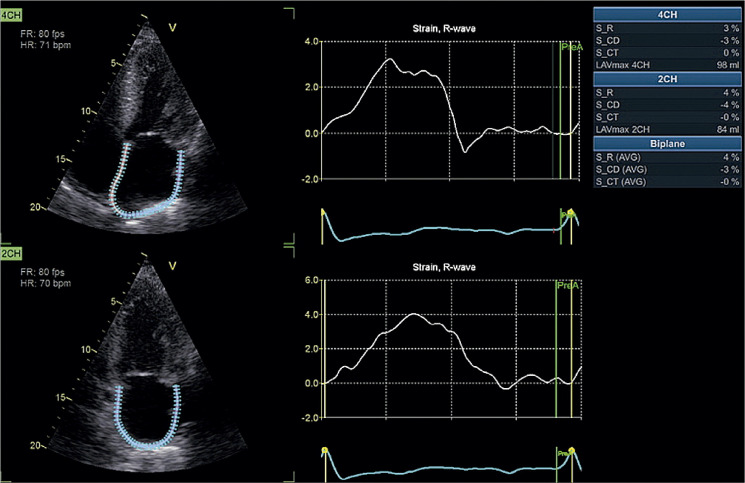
Amiloidose AL. Strain bidimensional do átrio esquerdo (AE), com análise das janelas apical 4 câmaras e apical 2 câmaras demonstrando importante redução do valor do strain de AE biplanar (strain reservatório = 4%).
